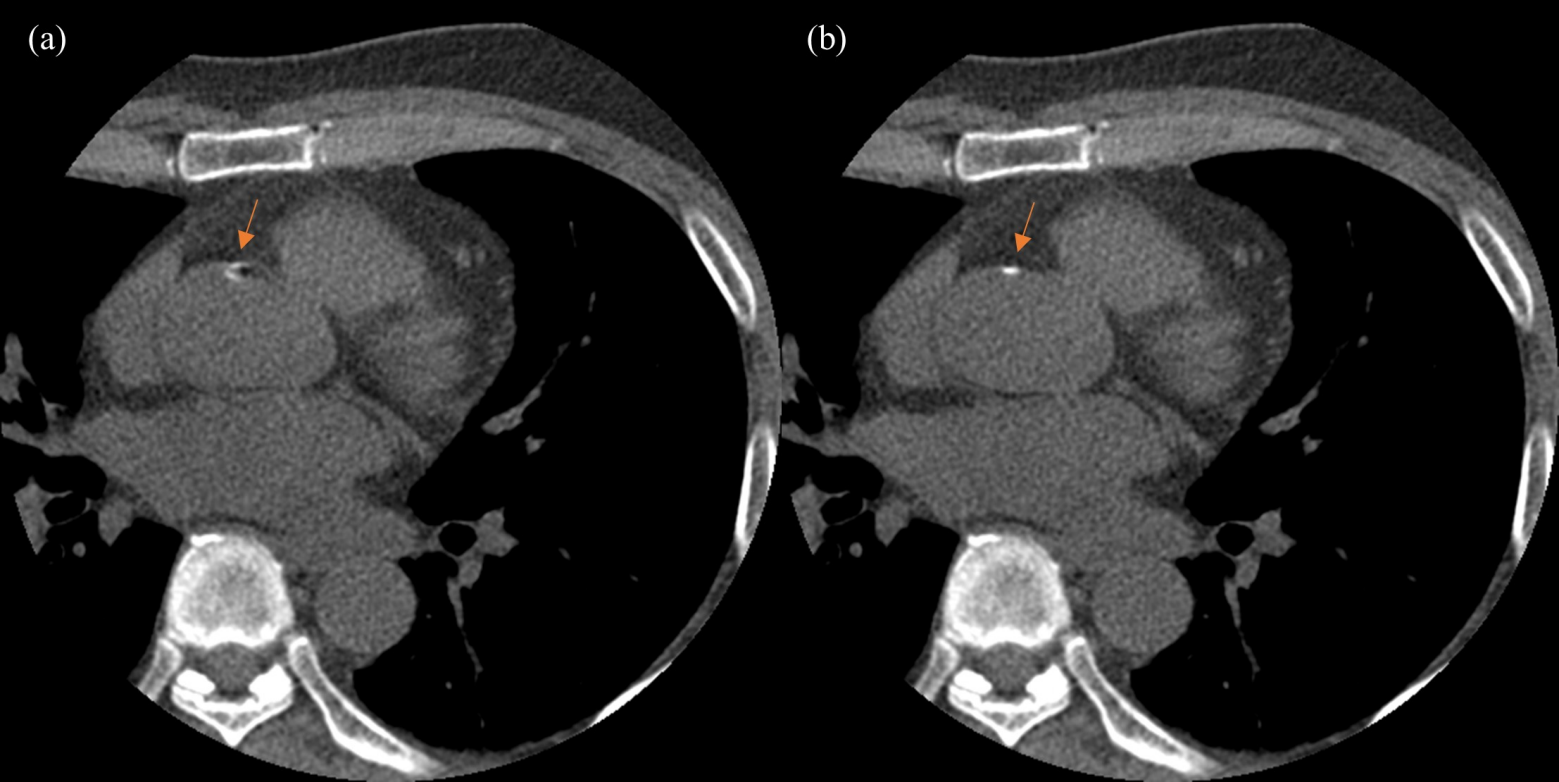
Performance of SCULLI in non-contrast cardiac CT scan (95 bpm, Case C). (a) FBP at 40% R-R shows a calcified region in the ascending aorta with motion artifacts. (b) SCULLI shows the region without motion artifact despite the higher heart rate of 95 bpm (L/W 100/700).
